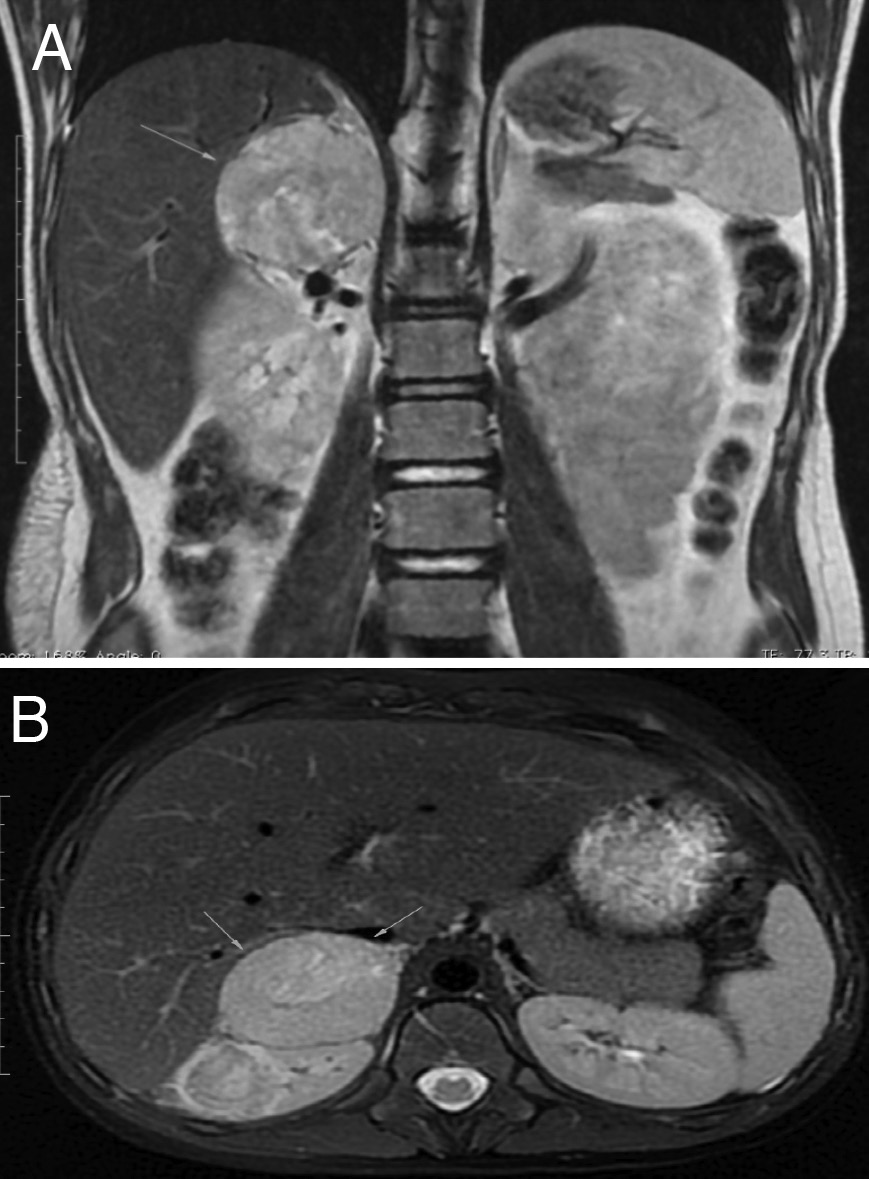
A, B) Abdominal magnetic resonance imaging findings: 41x46x45 mm solid mass lesion (green arrows), which had heterogeneous but diffuse contrast enhancement, was located between the liver and anterior upper pole of right kidney, displaced the liver to anterior
